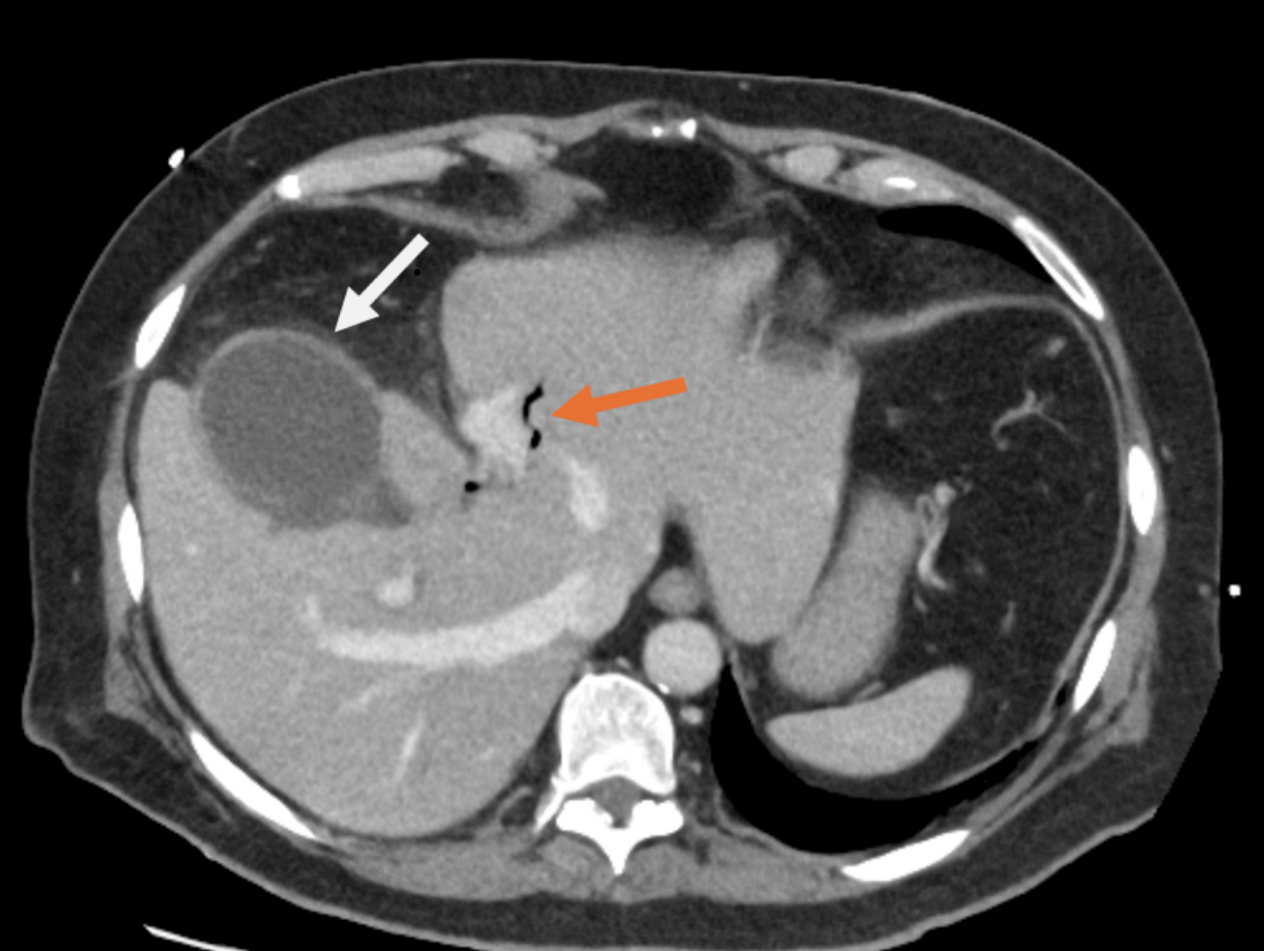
Axial slice of a CT abdomen and pelvis with contrast (portovenous phase). The image shows a distended gallbladder with mural thickening and pericholecystic fluid (white arrow) and a longstanding pneumobilia (orange arrow).
